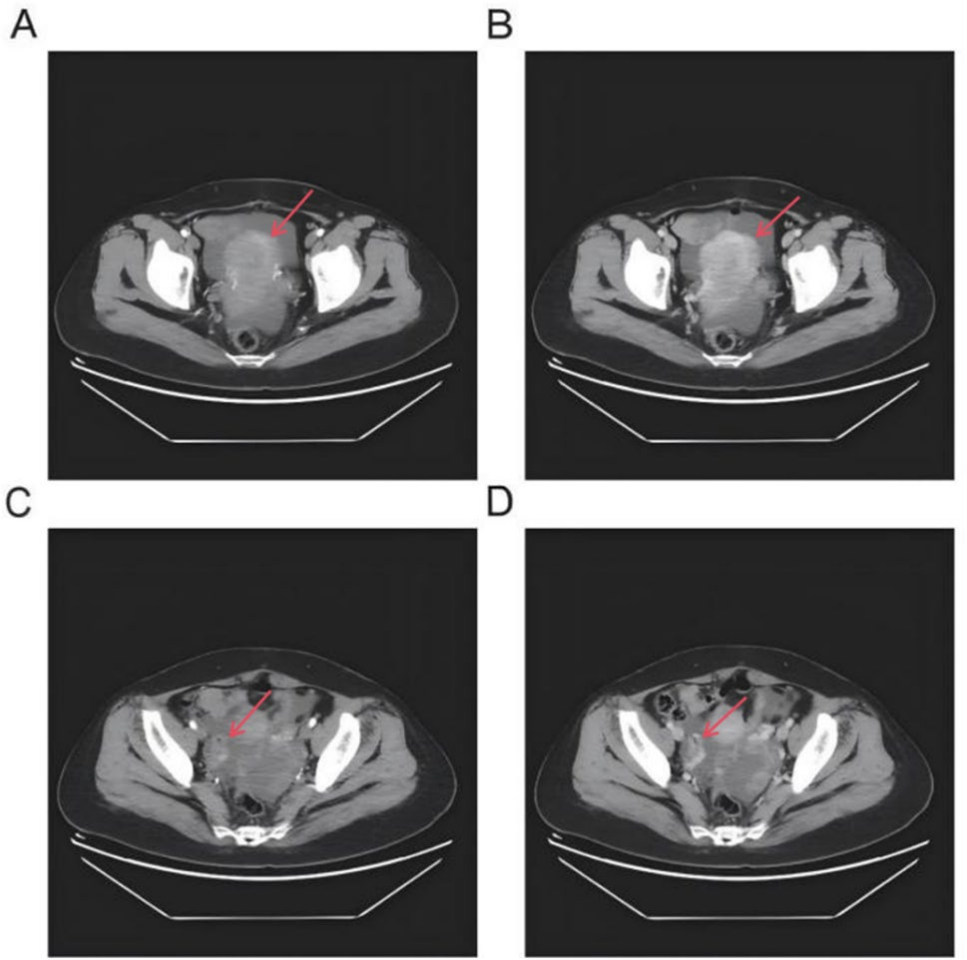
Representative pre-operative abdominopelvic computed tomography (CT) images showing the uterine lesion (A-B) and the ovarian mass (C-D). Arrows indicate the primary tumors. The red arrows highlight the specific locations of the tumors in both the uterine and ovarian regions
